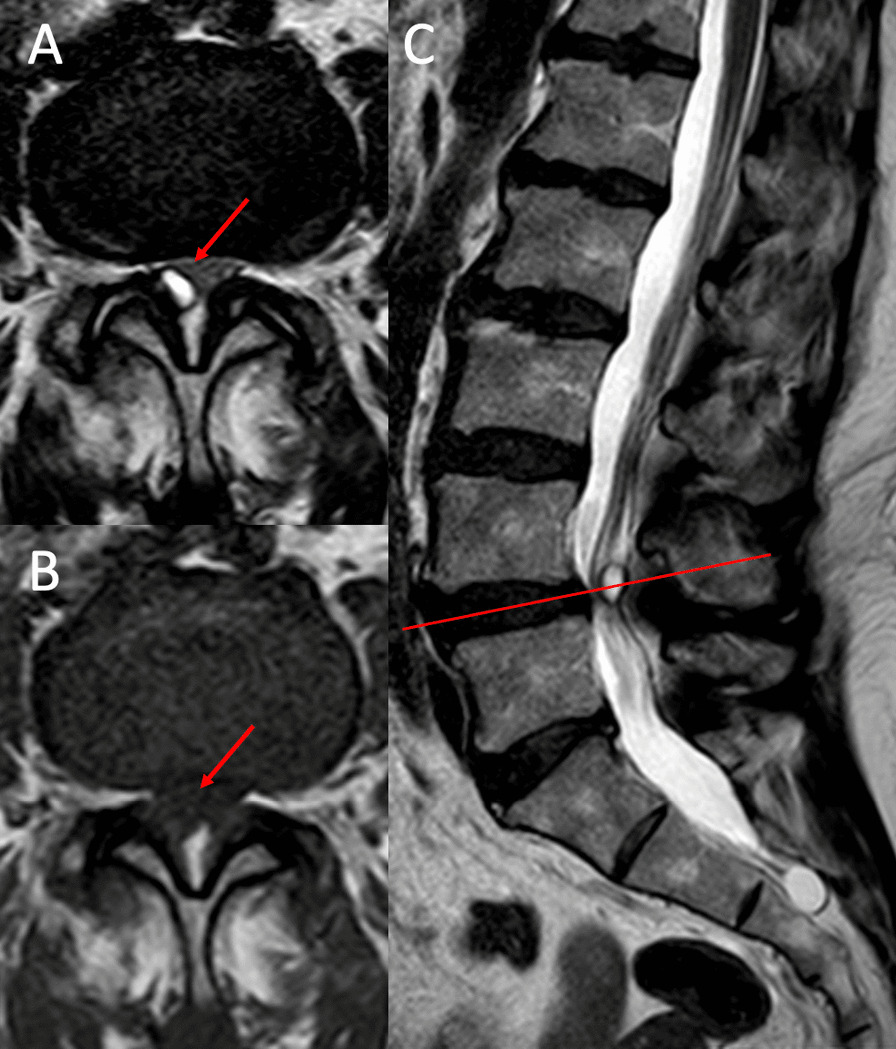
Synovial cyst at L4–L5 level in MRI. A Axial level cut in T2 sequence. B Axial section in T1 sequence. C Sagittal section in T2 sequence
